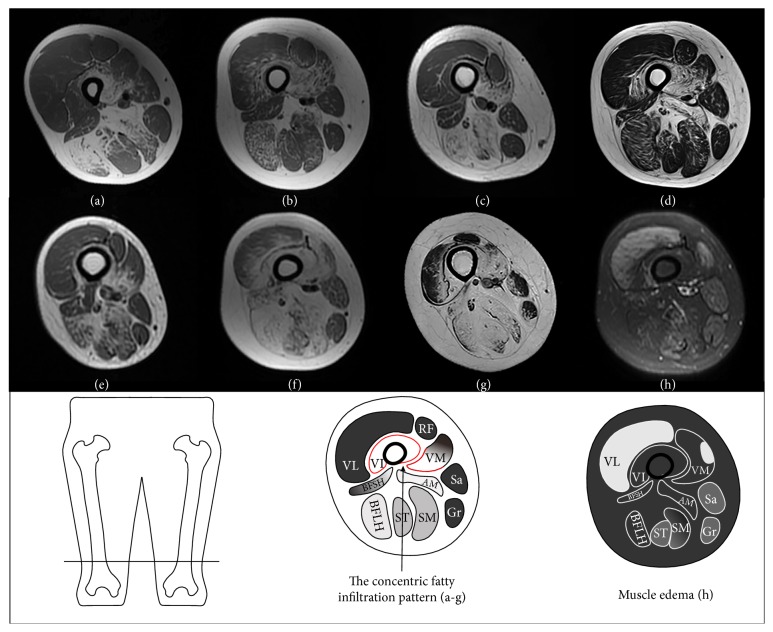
Axial T1-weighted images of the right thigh for patients 4–10 (a–g) showing a concentric fatty infiltration pattern around the distal femoral diaphysis and an axial short T1 inversion recovery image of the right thigh in patient 9 (h) showing relatively marked edematous changes in the thigh muscles.
